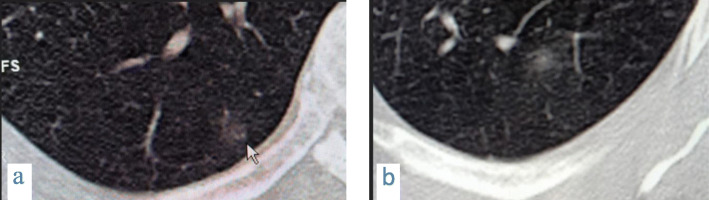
A 56-year-old male patient with COVID-19, CT chest magnified axial images showing (a) small fairly rounded subpleural ground glass opacity in the right lower lobe (arrow) and (b) a left lower lobe fairly rounded peripheral small ground glass opacity
